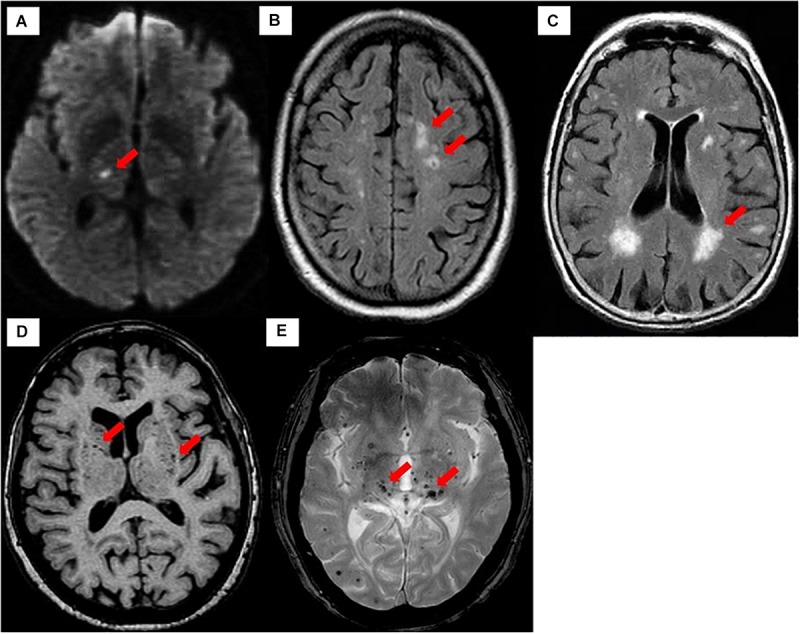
Neuroimaging classification of CSVD based on STRIVE. (A) Recent small subcortical infarct on DWI (arrow). (B) Lacune on FLAIR (arrow). (C) WMHs on FLAIR (arrow). (D) Perivascular spaces on T1-weighted imaging (arrow). (E) Cerebral microbleeds on T2^∗^-GRE (arrow).
